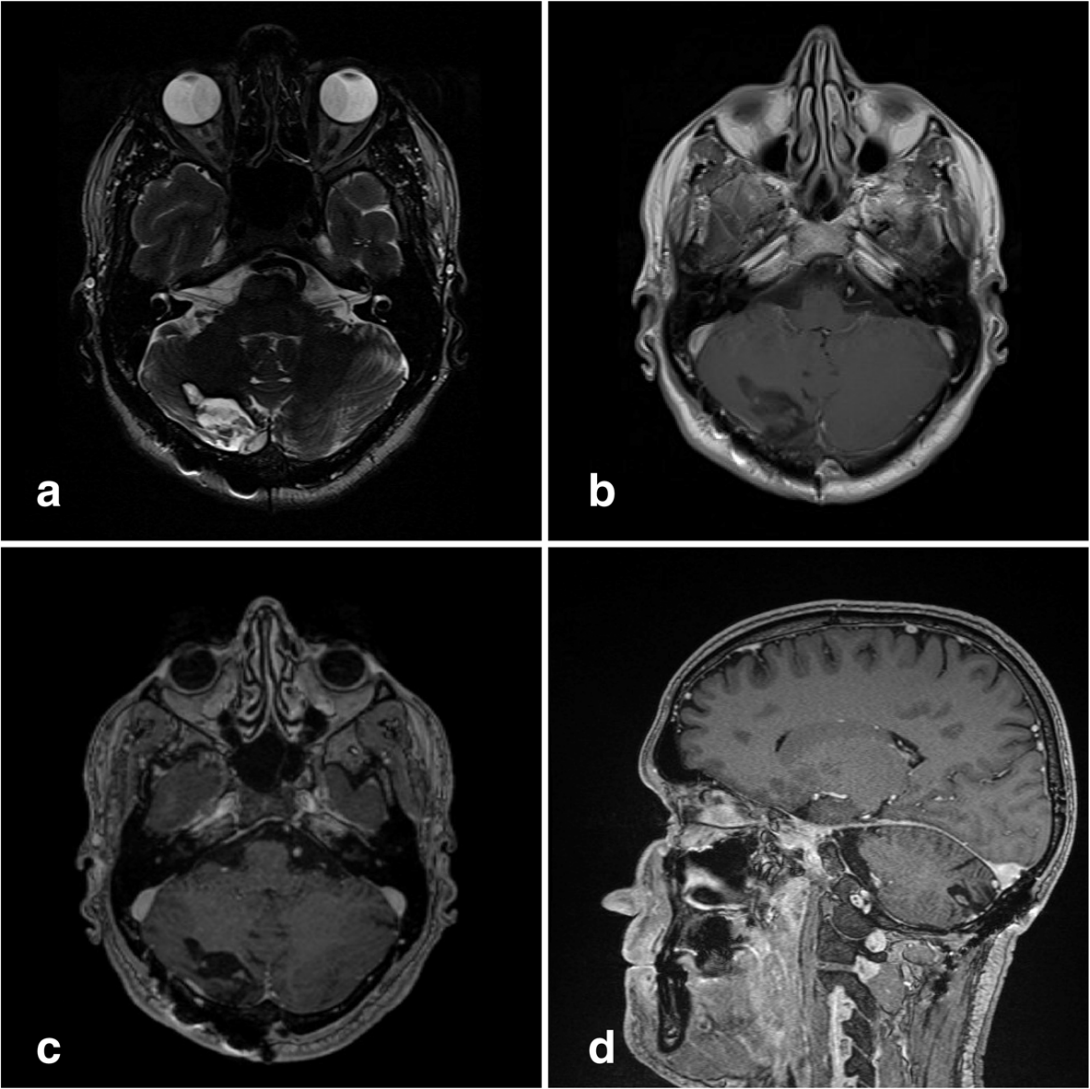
Postoperative magnetic resonance imaging 15 months after surgery shows no recurrence of the tumor on T2-weighted imaging (a) and on T1-weighted imaging (b). No contrast enhancement can be seen on T1-weighted images with contrast enhancement (c + d)
